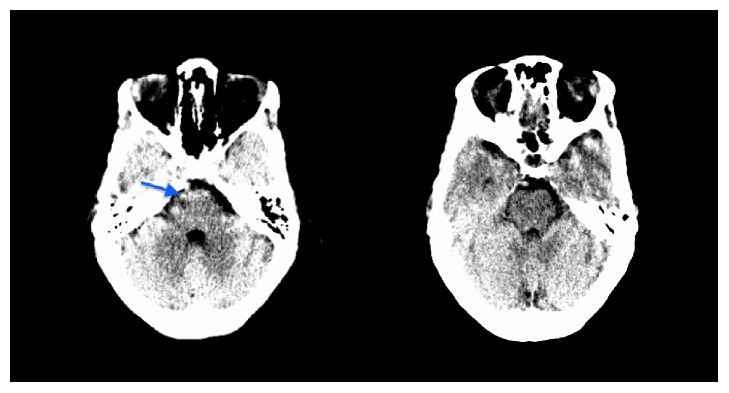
CT scan of the head on admission. Imaging shows no gross acute intracranial abnormality, except for mild bifrontal cerebellar atrophy and a 0.5 × 0.3 cm focal hypodense signal in the right pons (arrow), defined as beam hardening artifact.
